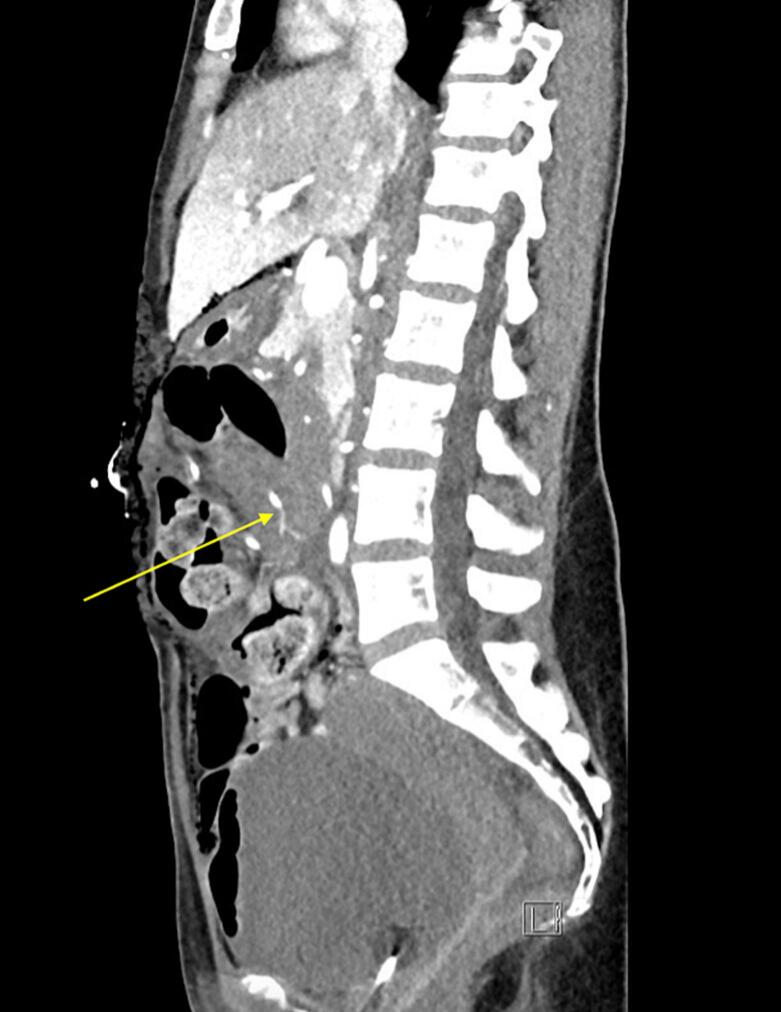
Computed tomography angiography demonstrating 5 mm pseudoaneurysm from irregular arterial branch of middle colic artery (arrow), with associated surrounding haemoperitoneum.
